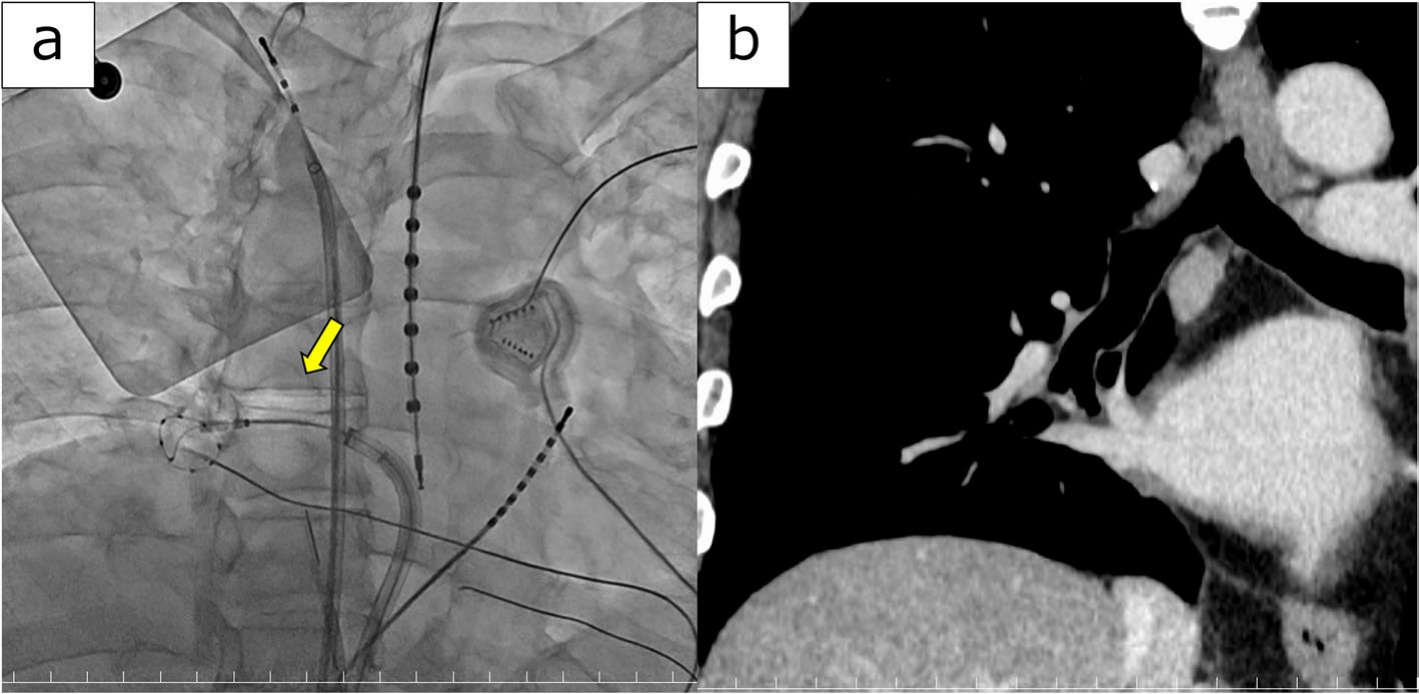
a Findings of cryoablation: A cryoballoon is positioned in the right inferior pulmonary vein (arrow). b Preoperative chest CT findings: No obvious abnormalities such as stenosis or wall thickening are observed in the inferior pulmonary vein
